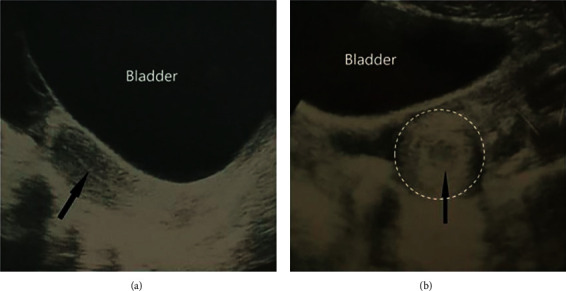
(a) On ultrasound, sagittal view of the pelvis shows the uterus with a normal uterine contour and normal echogenic endometrial line (arrow). (b) Axial view of the pelvis demonstrates a cross section of a mass-like lesion on the left side of the pelvic cavity, which was finally proved to be an accessory and cavitated uterine mass (arrow marks the endometrium).
